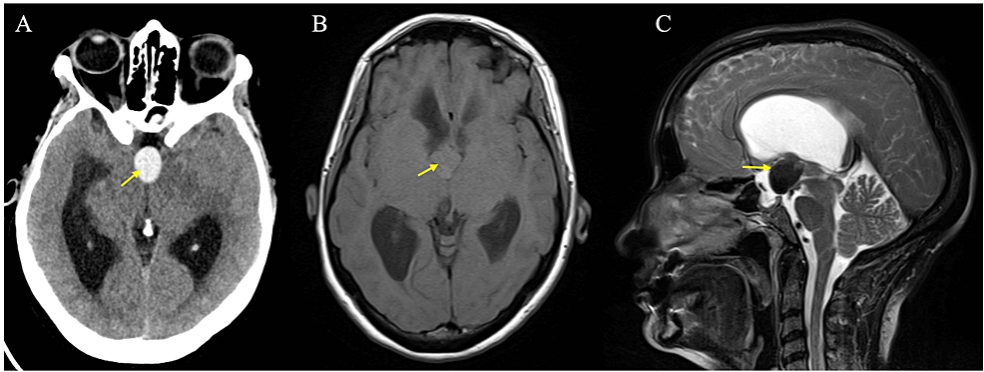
The axial CT scan shows a well-defined hyperdense third ventricular lesion at the level of foramina of Monro (A) (yellow arrow). In an MRI scan, the same lesion is isointense on T1 (B) (yellow arrow) and hypointense on T2 (C) (yellow arrow) with supratentorial hydrocephalous. All the features indicate a diagnosis of a colloid cyst.
